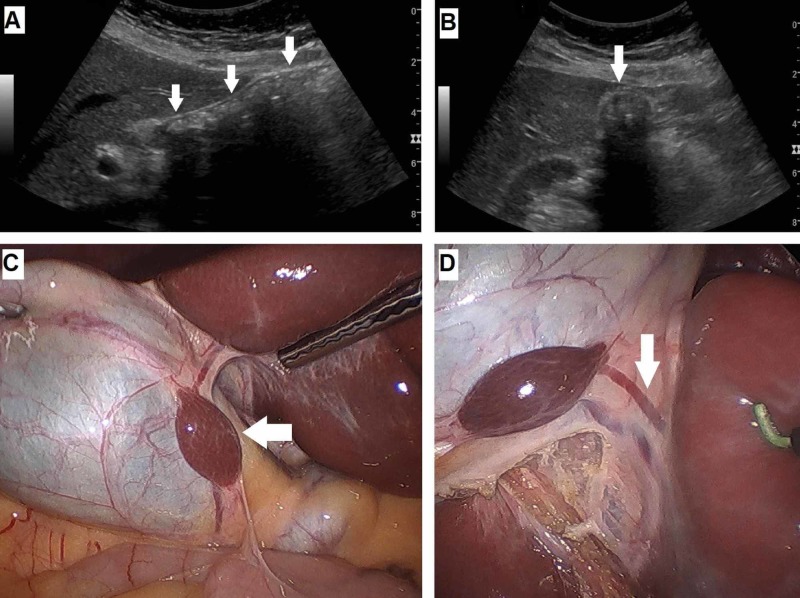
Ultrasound images and intraoperative findings A, Sagittal and B, Transverse ultrasonography images of multiple gallstones represented by reflective echogenic foci within gallbladder lumen with prominent posterior acoustic shadowing (arrows). C, Intraoperative laparoscopic view of the gallbladder retracted over the anterior edge of the liver, revealing an encapsulated accessory liver lobe on the serosal surface of the anteromedial gallbladder wall (arrow). D, Arrow points at a separate vascular pedicle draining the accessory liver lobe well visualized after the mobilization and dissection of the gallbladder from its liver bed.
